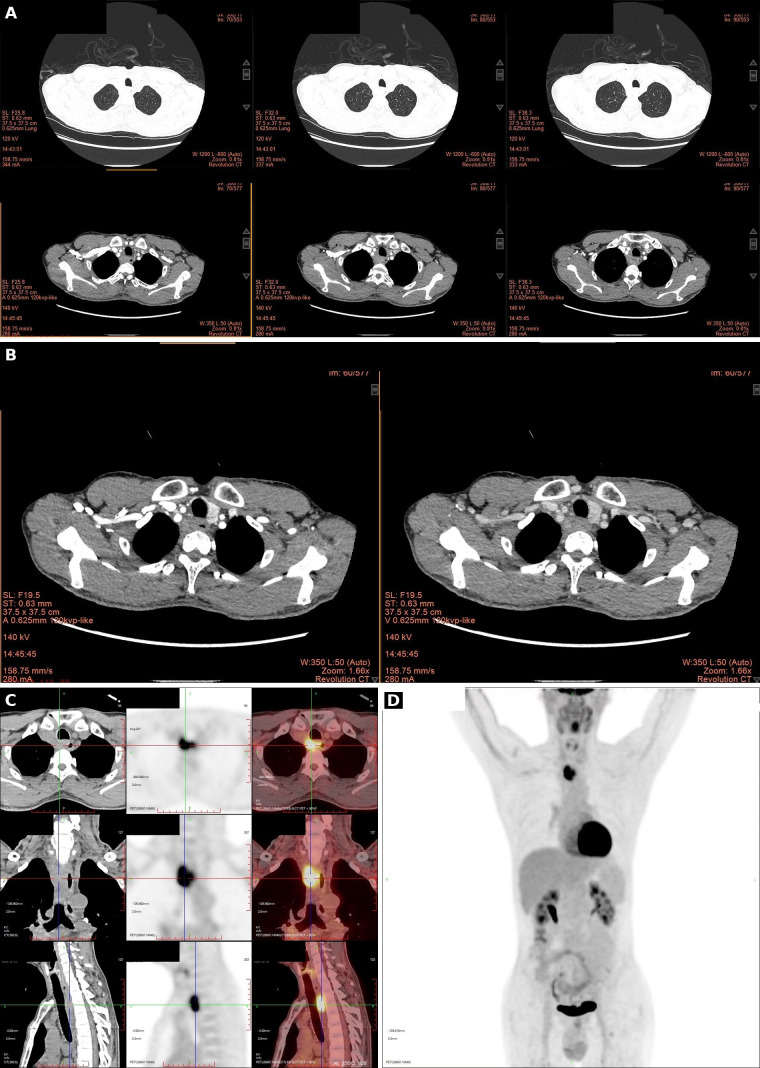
Pre-treatment diagnostic imaging. (A) Contrast-enhanced chest CT at contiguous levels (top: lung window; bottom: mediastinal window) showing an ill-defined soft-tissue lesion posterior to the trachea, within the tracheoesophageal groove of the superior mediastinum, with a poorly defined interface with the right lateral esophageal wall. (B) Magnified mediastinal-window images on the arterial phase (left) and venous phase (right), demonstrating heterogeneous enhancement of the mass and its close relationship to the adjacent great vessels. (C) Axial CT, PET, and fused [18F]FDG PET/CT images demonstrating intensely elevated FDG uptake (SUVmax 16.7) in the lesion, with no abnormal uptake in regional lymph nodes. (D) Whole-body maximum intensity projection (MIP) image showing a solitary mediastinal focus of intense uptake and no abnormal uptake elsewhere to suggest distant metastasis (physiological uptake is seen in the brain, myocardium, kidneys, bowel, and bladder).
